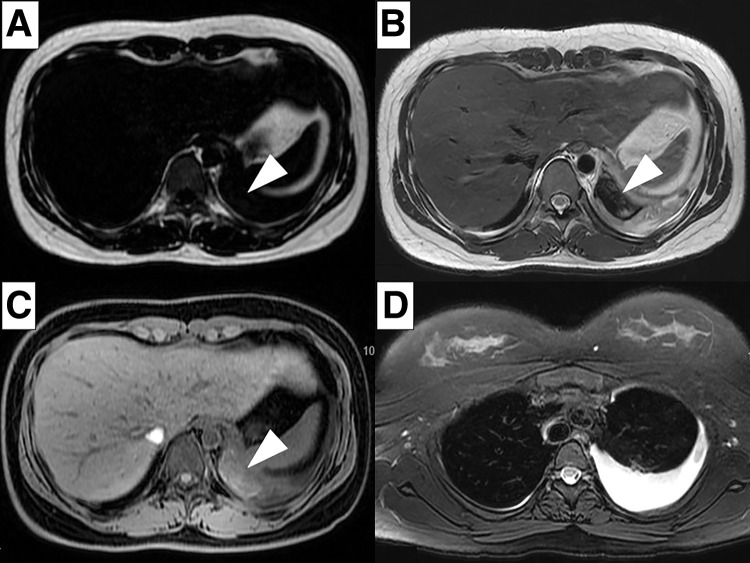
(A, B) MRI showing a low signal intensity both on the T1- and T2-weighted images (arrowheads). (C) Fat-suppressed T1-weighted images showing a slightly high signal intensity (arrowhead). (D) Fat-suppressed T2-weighted images showing fluid collection in the left pleural cavity.
